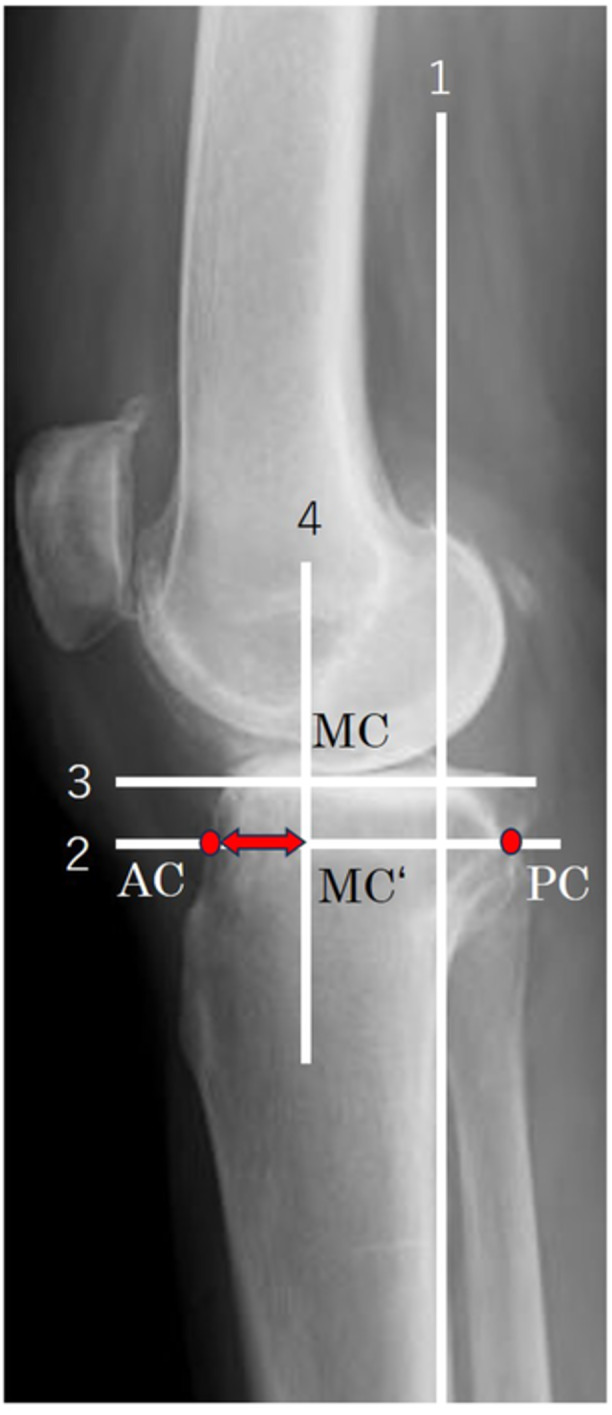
Contact point measurement on standing lateral radiographs. Line 1: a line parallel to the posterior tibial cortex. Line 2: a line 7 mm below the medial tibial plateau and perpendicular to Line 1. Line 3: a line parallel to Line 2 and tangent to the most distal point of the medial condyle (MC). Line 4: a line perpendicular to Line 3 passing through points MC and Line 2. MC′: the crossing between Lines 2 and 4. AC–MC′: the distance between the anterior cortex (AC) and the posterior cortex (PC) of the tibia on Line 2. AC‐PC: distance between AC and PC. The tibiofemoral contact point is calculated as (AC–MC′/AC–PC).
